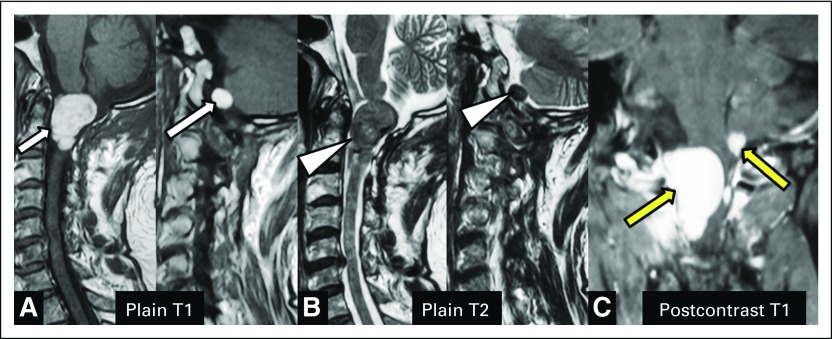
Images obtained before the second surgery (2012). Magnetic resonance imaging showed well-defined intradural extramedullary lesions at the cervicomedullary junction. On the plain T1-weighted sagittal view (A), the mass was hyperintense (white arrows); on the plain T2-weighted sagittal view (B), it showed hypo-intense signal intensity (arrowheads). The postcontrast T1-weighted coronal view (C) showed intense postcontrast enhancement (yellow arrows). The imaging findings described were diagnostic for melanoma.
